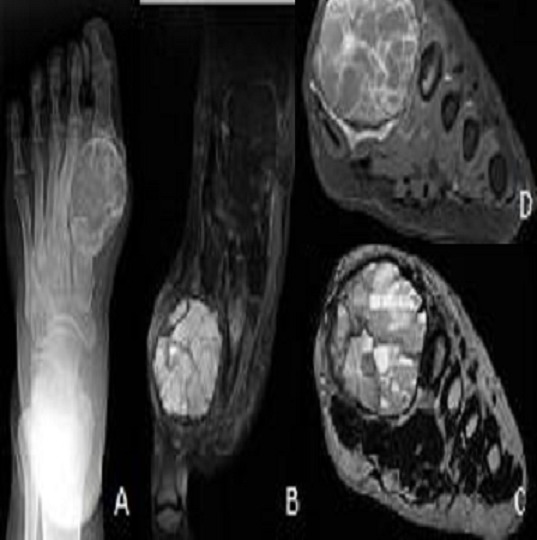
Radiography (A) showing a multiloculated osteolytic lesion of the metatarsal very limited. Cuts sagittal T1-weighted MRI (B), axial T2 (C) and FAT SAT T1 after contrast (D) showing an enhancement of intralesional walls with liquid level
